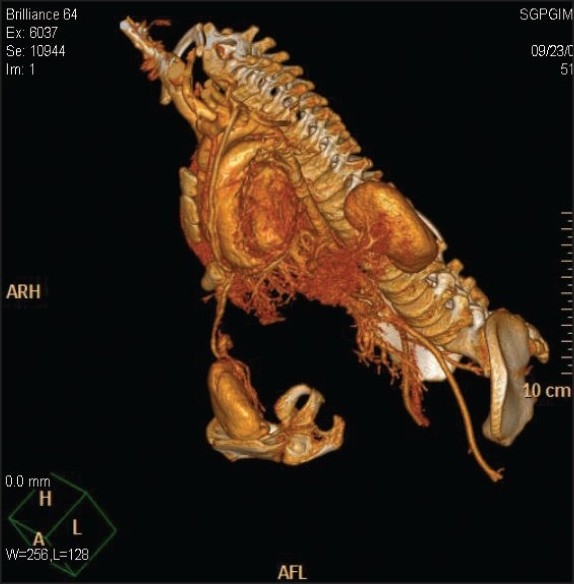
3D-reconstructed CT image showing extra kidney supplied by the internal mammary artery of child
